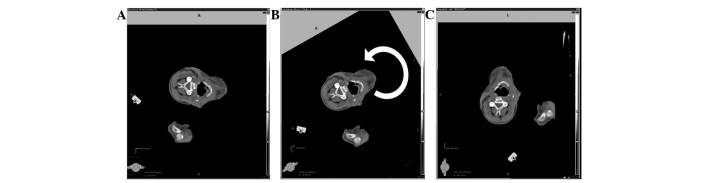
Rotation of axial slices for radiotherapy planning target volume contouring. (A) CT image in lateral position as used for radiation treatment. (B) Rotation of CT image. (C) Rotated CT image from A.
